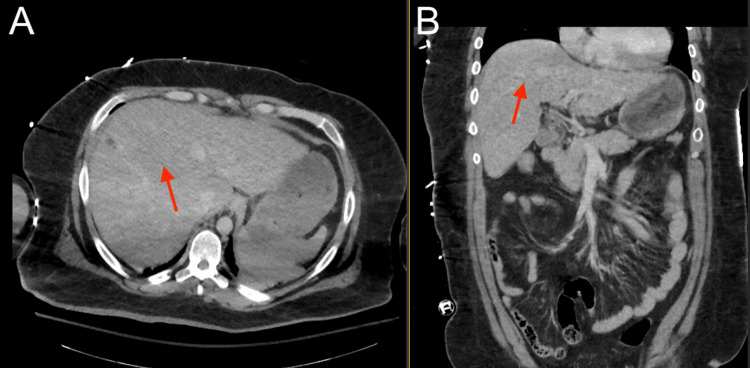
Abdominal CT showing suspected chronic liver disease (red). (A) Axial view; (B) Coronal view CT: computed tomography
